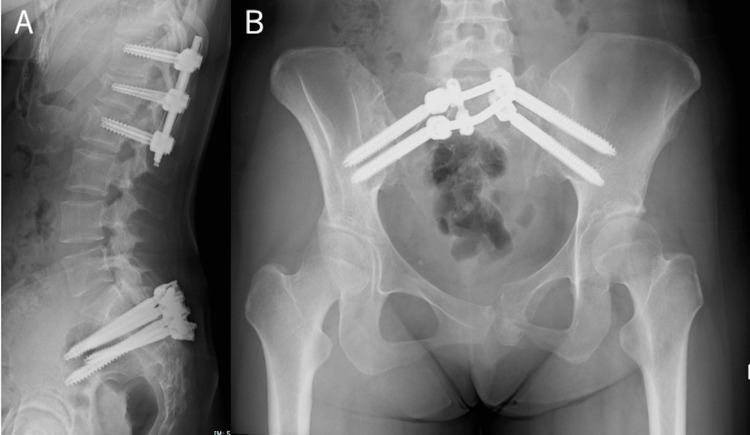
Postoperative imaging findings. (A) Lateral X-ray view after the surgery; posterior fixation was performed at the T12–L2 level and pelvis. (B) Frontal X-ray view of the pelvis after the surgery; the pubic symphysis is mildly reduced.
